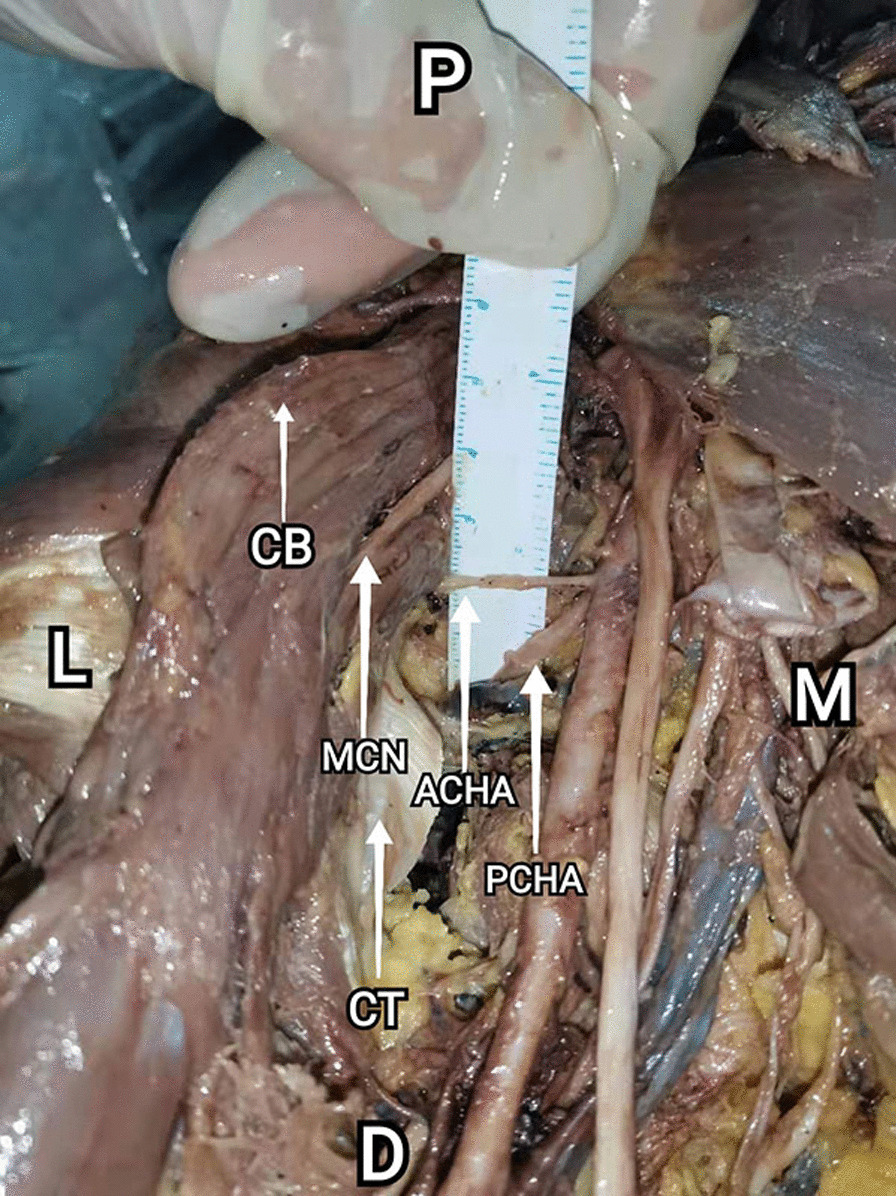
Inferior aspect of axilla (Right) (P: proximal D: distal M: medial L: lateral). CT: Conjoint tendon of the latissimus dorsi and teres major muscles; ACHA: Anterior circumflex humeral artery; PCHA: Posterior circumflex humeral artery; MCN: Musculocutaneous nerve; CB: Coracobrachialis. Structures at risk: ACHA, PCHA, musculocutaneous nerve. The interval between ACHA and PCHA is the area that suitable for the placement of the medial plate
